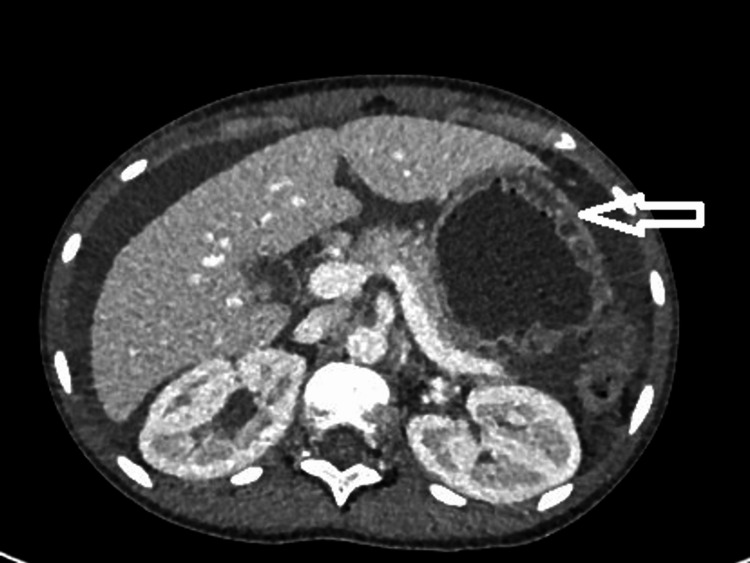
Contrast-enhanced CT of the abdomen and pelvis in the axial plane revealing gastric antral vascular ectasia (“watermelon stomach”) The stomach wall (arrow) has scalloped and prominent antral folds that radiate to the pylorus. The internal vasculature of the stomach is ectatic and enlarged, which follows the fold pattern to congregate towards the antrum. This gives a characteristic “watermelon stomach” appearance. This finding in the context of an inflamed bowel is often associated with autoimmune-related colitis.
